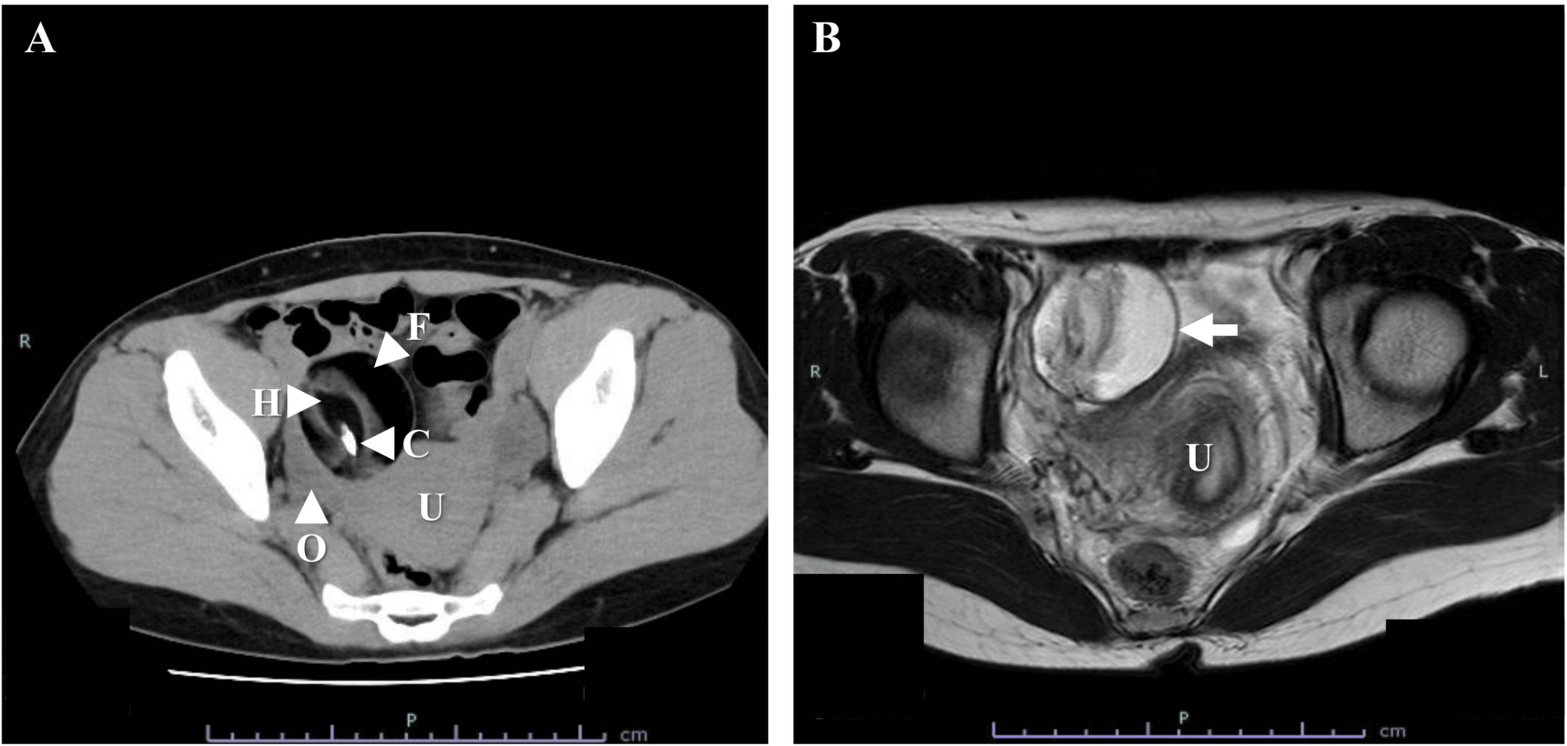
CT and MRI findings of the pelvic tumor (A) CT revealed a 5-cm, well-circumscribed, unilocular cystic lesion in the right adnexal region. The lesion contained fatty fluid, calcification, and a hairball. (B) MRI (T2-weighted images) showed a 5-cm, well-circumscribed, high-intensity mass in the right adnexal region (arrow). Its continuity with the right ovary was unclear. No ascites was observed. C: calcification; F: fatty fluid; H: hairball; O: right ovary; U: uterus
